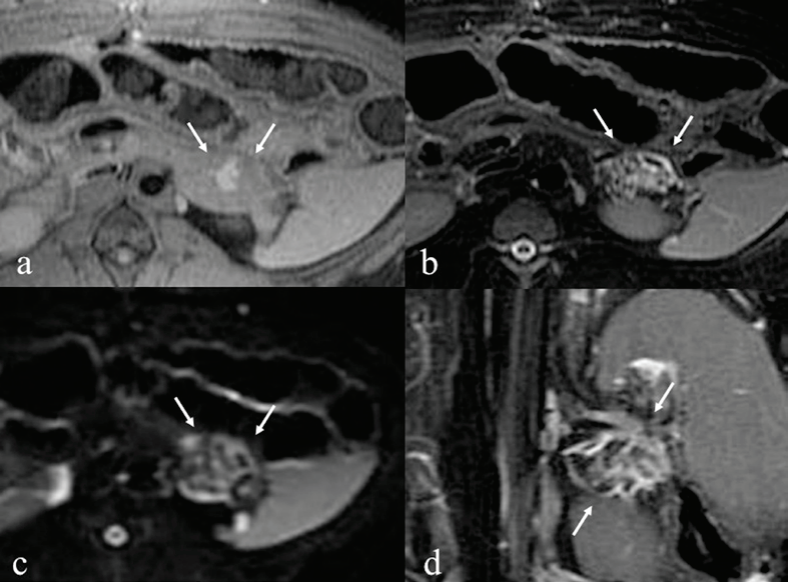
MRI immediately after injection with 1.0 mL ethanol. a: T1-weighted image shows small high signal areas measuring 12 x 7 mm in the pancreatic tail. b: T2-weighted image shows linear high signal areas measuring 35 x 32 mm. c: DWI image shows rounded speckles high signal areas. d: T2 coronal image.
